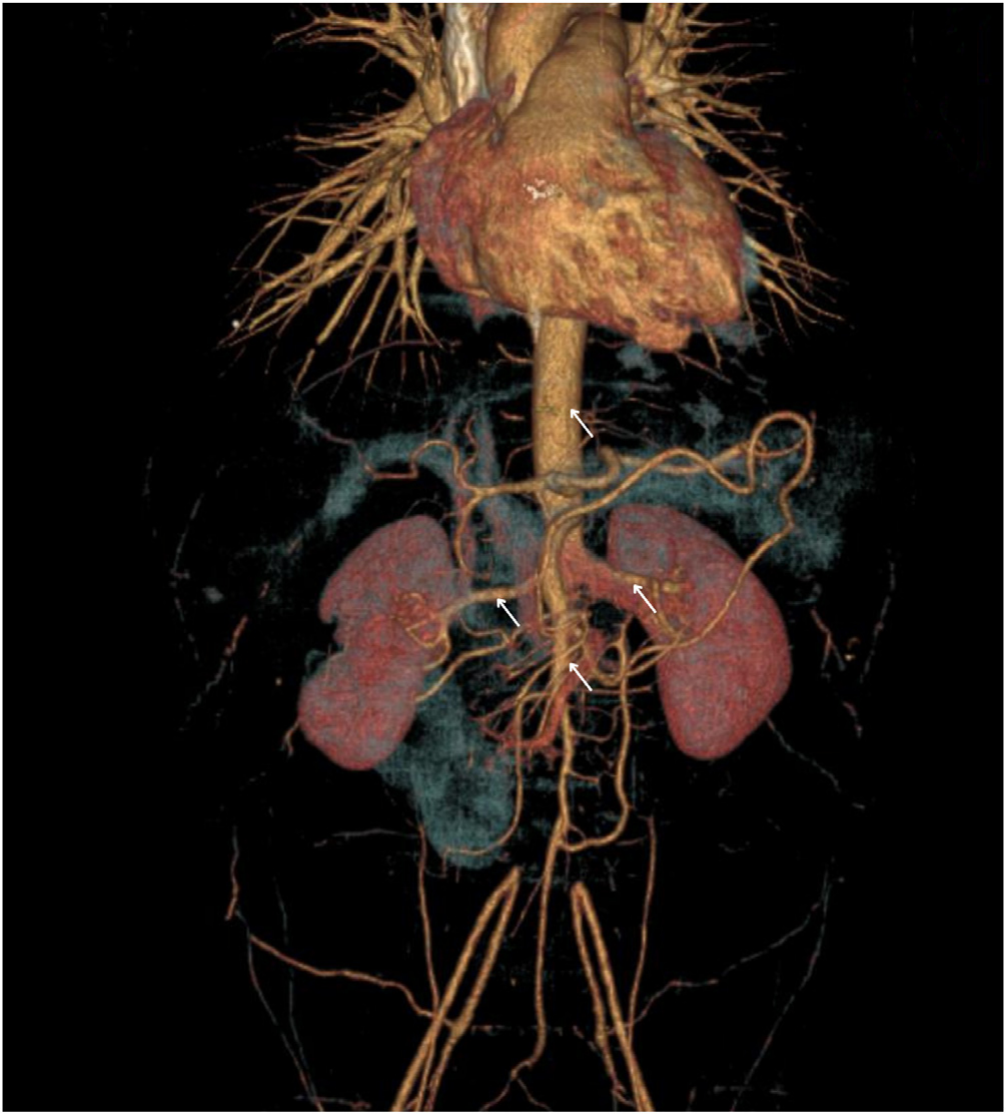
3D reconstruction of chest-abdomen CTA showing extensive large-vessel involvement (arrow) typical of Type V Takayasu arteritis.
